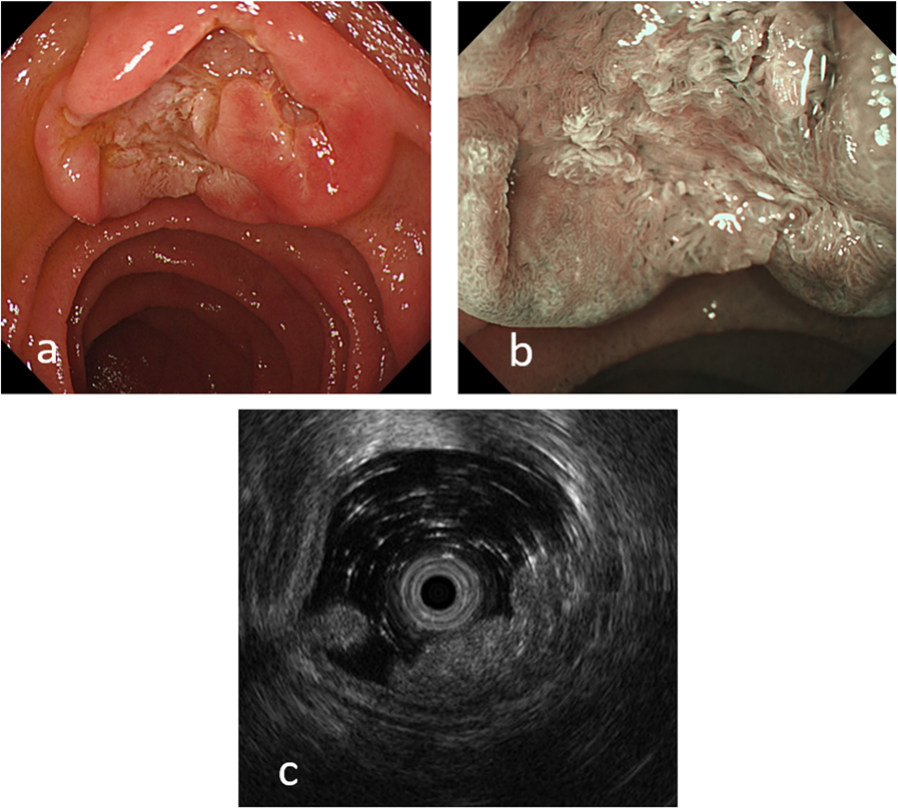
a An endoscopic picture showing a wide-based sessile submucosal tumor-like mass with depression at the top of the lesion. b Narrow banding image with magnification showing non-irregular microvessel or mucosal structure. c Endoscopic ultrasound images showing a heterogeneously hyperechoic mass with several anechoic lesions located predominantly in the mucosa
